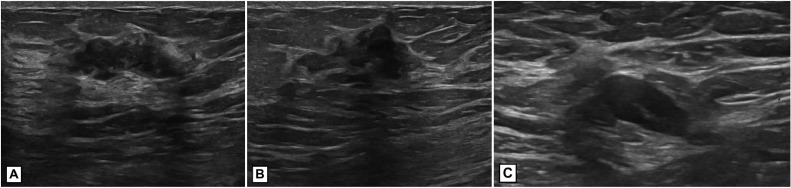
Ultrasound of left breast and axilla. Two irregular vascular masses seen on ultrasound: a 2.6 cm lesion at 1 o'clock, 12 cm from the nipple areola complex (A) and a 1.3 cm lesion at 2 o'clock, 2 cm from the nipple areola complex (B). Prominent axillary lymph node with 4 mm of cortical thickening (C).
